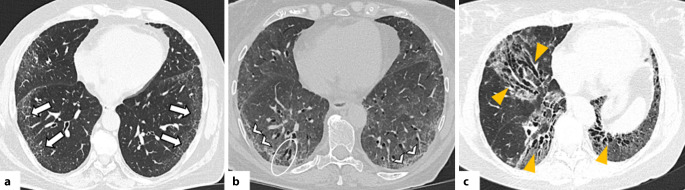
Axiale Aufnahmen der hochauflösenden Computertomographie (HRCT): Muster der nichtspezifischen interstitiellen Pneumonie (NSIP) bei Patienten mit systemischer Sklerose. a Milchglasverdichtungen, überwiegend in den peripheren Anteilen der Unterlappen, mit relativer subpleuraler Aussparung (*weiße Pfeile*). b Diffuse Milchglasverdichtungen und bilaterale periphere Retikulationen (*weiße Pfeilköpfe*), begleitet von Traktionsbronchiolektasen (*weißer Kreis*). c Fibrotischer NSIP-Subtyp: Milchglasverdichtungen in Mittel- und Unterlappen begleitet von zentralen Traktionsbronchiolektasen (*gelbe Pfeilköpfe*), die zentraler gelegen sind als beim Muster der gewöhnlichen interstitiellen Pneumonie (UIP). Zusätzlich zeigt sich in den Unterlappen ein ausgeprägter Volumenverlust
